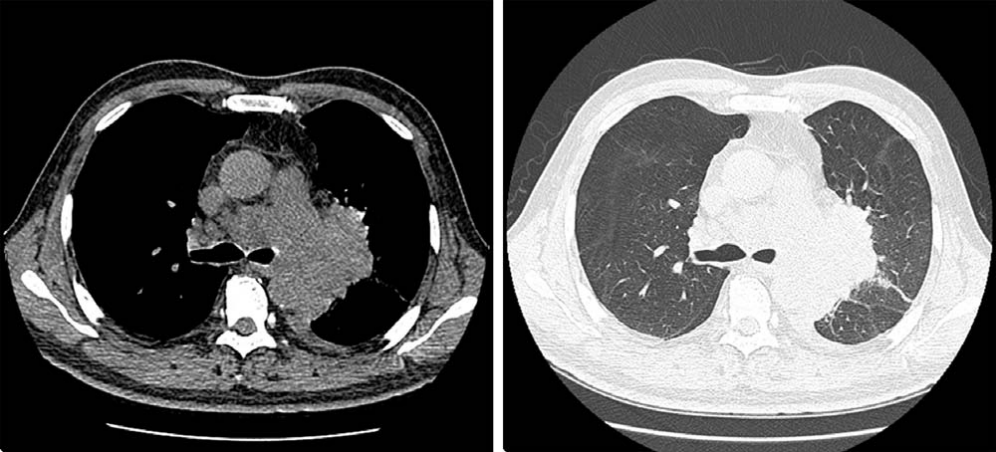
CT scan shows bronchial soft tissue shadow on the posterior segment of the left upper lung apex, unclear borders, obstruction of the posterior segment of the upper lung apex, and enlarged lymph nodes in the mediastinum.
